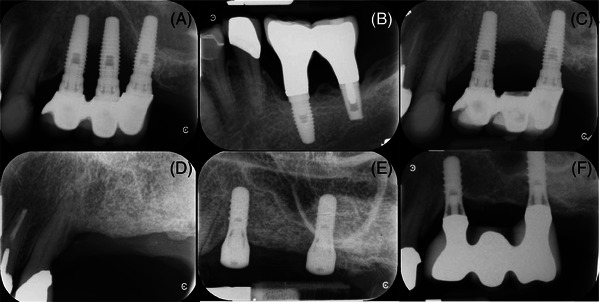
Radiographic series for patient 2. (A) In 2014, 20 years after placement, the patient presented with an ailing 3‐IS three‐unit FDP; (B) the opposing 2‐IS three‐unit FDP (Nobel Replace CC, Endopore) was healthy; (C) the middle implant of the 3‐IS three‐unit FDP was removed while severe bone loss at the mesial and distal implants also were later removed (D); (E) after GBR, two implants (Straumann SP) were placed; (F) 3‐year recall of the retreatment.
